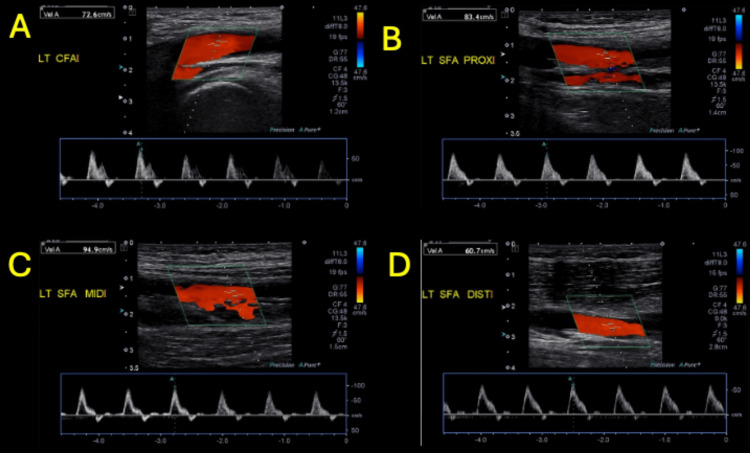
Left lower extremity arterial duplex ultrasound (part 1) Left common femoral artery (LT CFA) with triphasic waveform and peak systolic velocity 67 cm/s (A). Left superficial femoral artery proximal, middle, and distal (LT SFA PROX, MID, DIST) with triphasic waveform and peak systolic velocity 135 cm/s (B, C, D).
